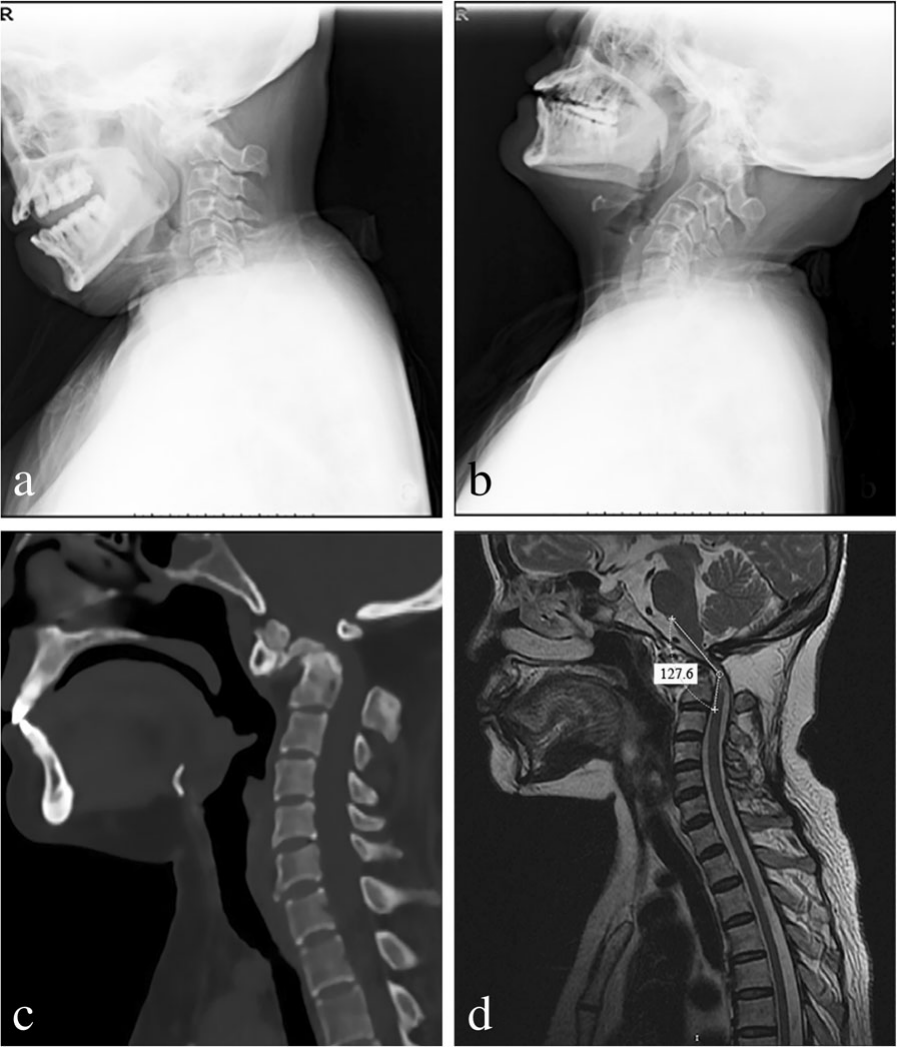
Case 6, 57-year-old female diagnosed with os odontoideum. a, b Flexion-extension radiographs showed no dynamic change of atlantodental interval (ADI) from flexion to extension. c Preoperative sagittal CT showing that the ADI is enlarged and the space available for the spinal cord (SAC) is decreased. d Sagittal MRI showed ventral compression of the spinal cord and a reduced CMA of 127.60°.
